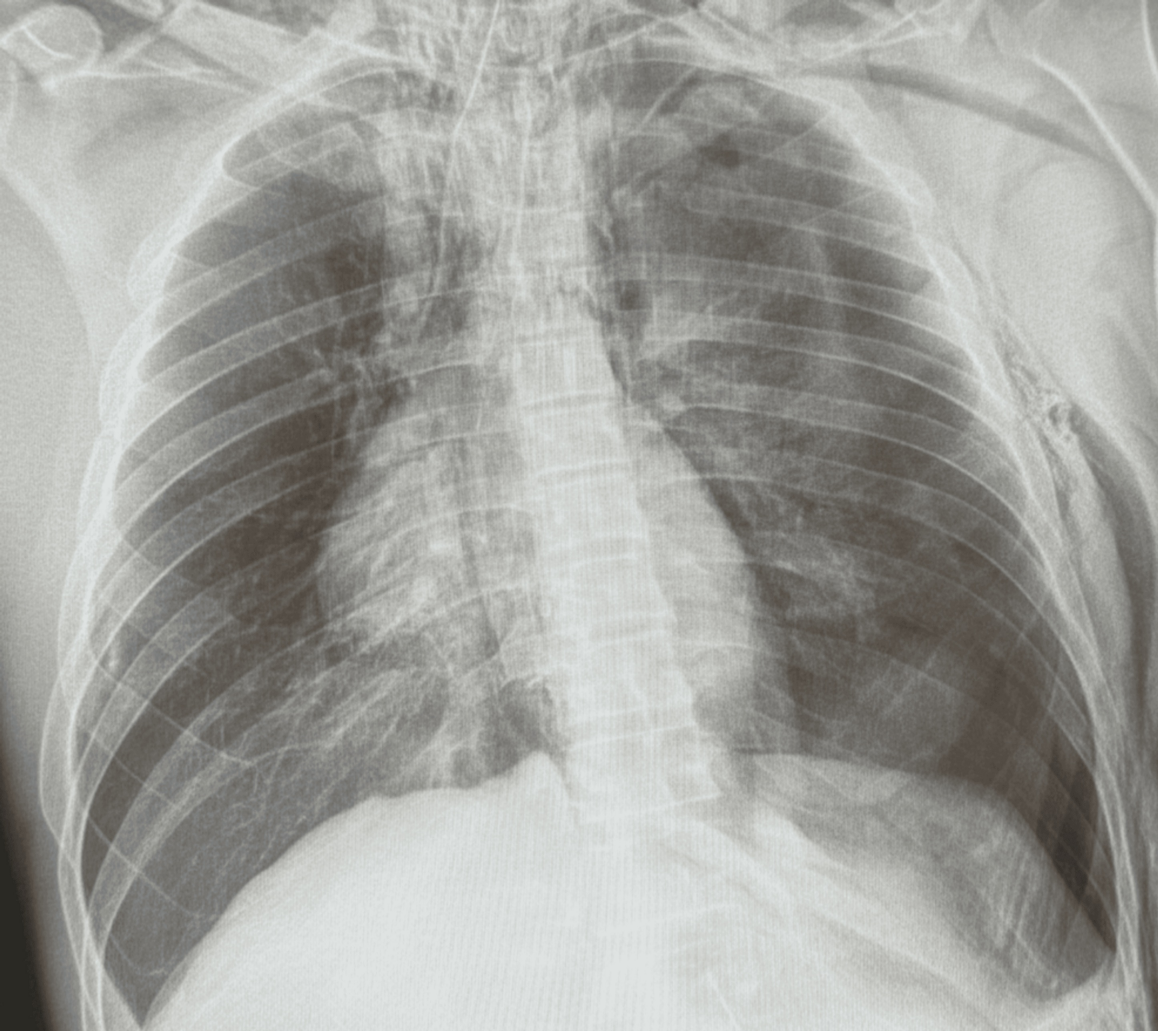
Anteroposterior chest radiograph obtained in the resuscitation room upon the patient’s arrival, demonstrating a left pneumothorax, subcutaneous emphysema, pneumomediastinum, and pneumopericardium.
